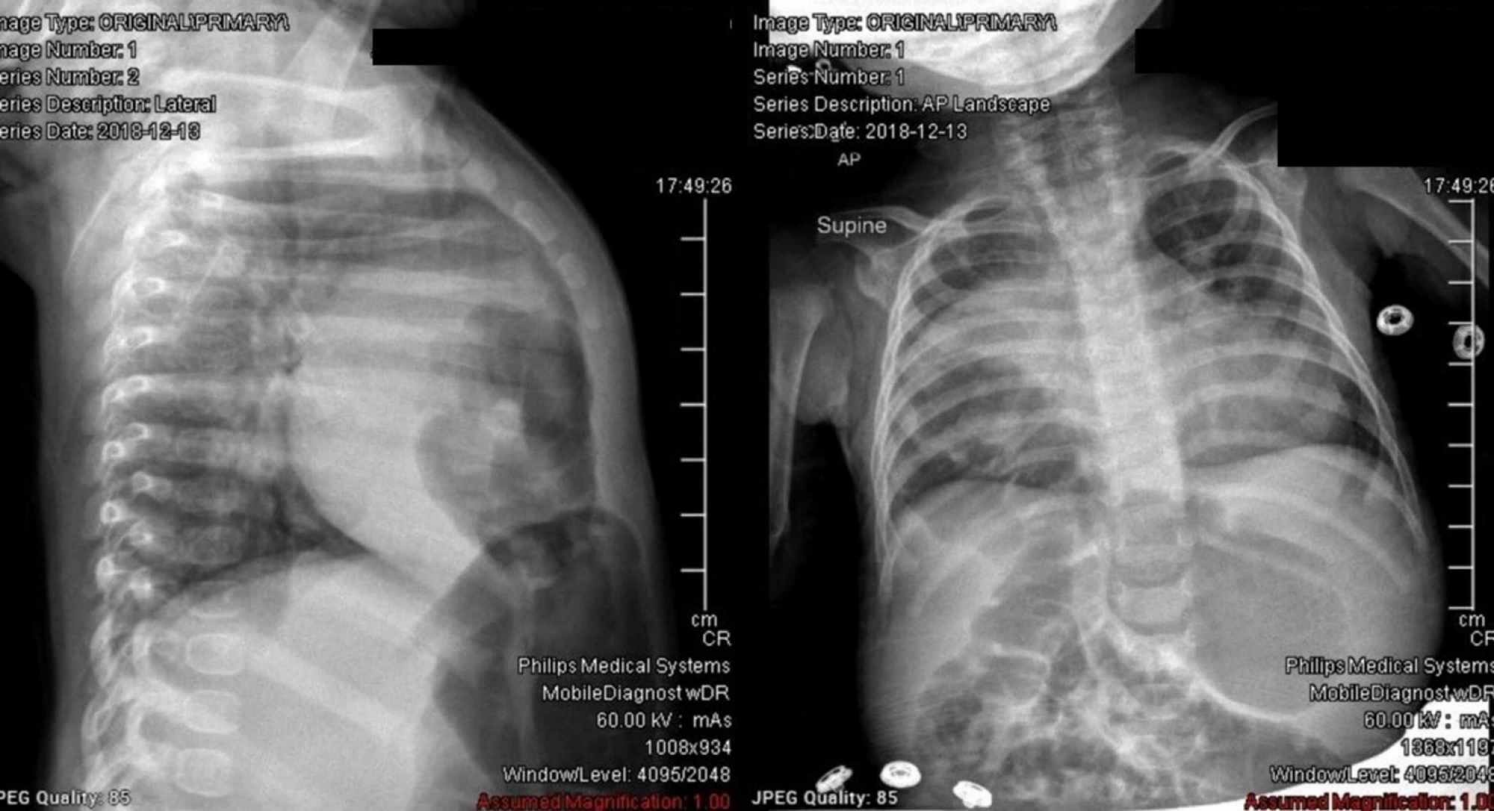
Lateral (on right) and anteriorposterior (on left) chest X-ray showing herniated colonic loops in the anterior right hemithorax consistent anterior right diaphragmatic hernia
